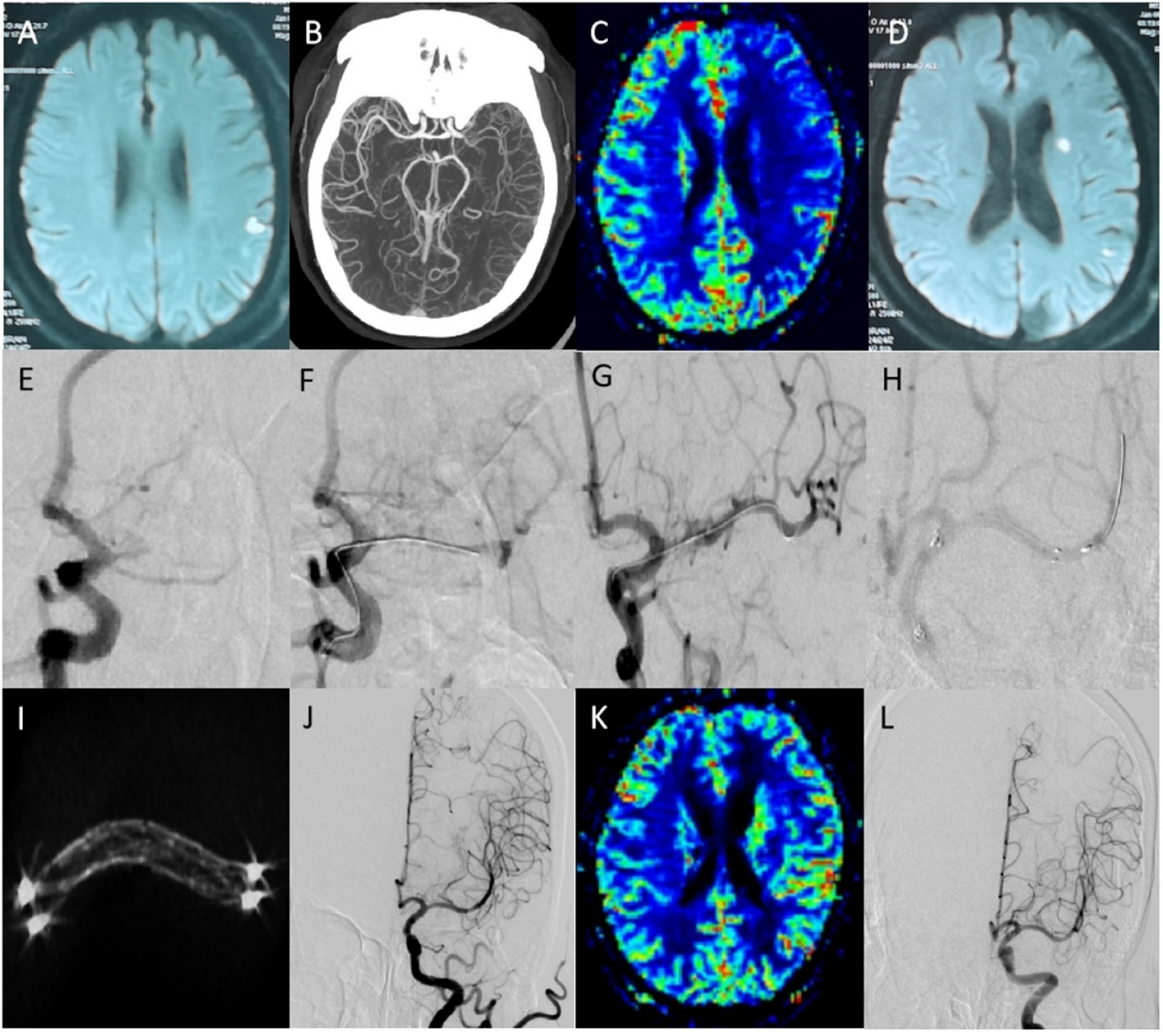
Clinical data of a low-risk patient. Male patient, 65 years old; chief complaint: right limb weakness with slurred speech for 21 days, relapsed for 3 days; past medical history: hypertension for 6 years; physical examination: the right limb muscle strength was grade 3, and the muscle tone was normal; the mRS score was 2. (A) Preoperative magnetic resonance imaging (MRI) shows acute cerebral infarction in the left cerebral parietal lobe. (B) Computed tomography angiography shows the M1 segment occlusion of the left middle cerebral artery. (C) Preoperative perfusion-weighted imaging (PWI) shows left cerebral hypoperfusion. (D) MRI after symptom recurrence shows left basal ganglia and parietal lobe acute cerebral infarction. (E) Preoperative digitally subtracted angiography (DSA) shows the left middle cerebral artery occlusion (occlusion length <5 mm, occlusion duration <3 months, atherosclerotic, no calcification, straight). (F) The micro-guide wire passed through the occluded segment during the operation. (G) The Gateway 2.0 × 10 mm balloon was used for accurate positioning and 4ATM expansion. (H) Neuroform 3.5 × 20 mm was ascended along the stent catheter, accurately positioned, and released. (I) The reconstructed stent is visible, and the stent is well expanded. (J) Postoperative anteroposterior angiography. (K) PWI shows that the bilateral perfusion was symmetrical, and the left cerebral perfusion was significantly improved. (L) Angiography was repeated 5 months later, which showed stent patency, and the mRS score was 0.
